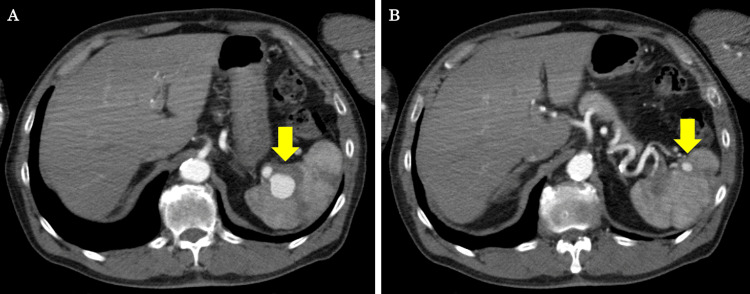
CECT findings. (A) A 25-mm dilated aneurysm was found at the distal end of the upper pole branch of the splenic artery (a yellow arrow). (B) A 10-mm aneurysm was found at the end of the lower pole branch of the splenic artery (a yellow arrow). CECT: contrast-enhanced computed tomography
